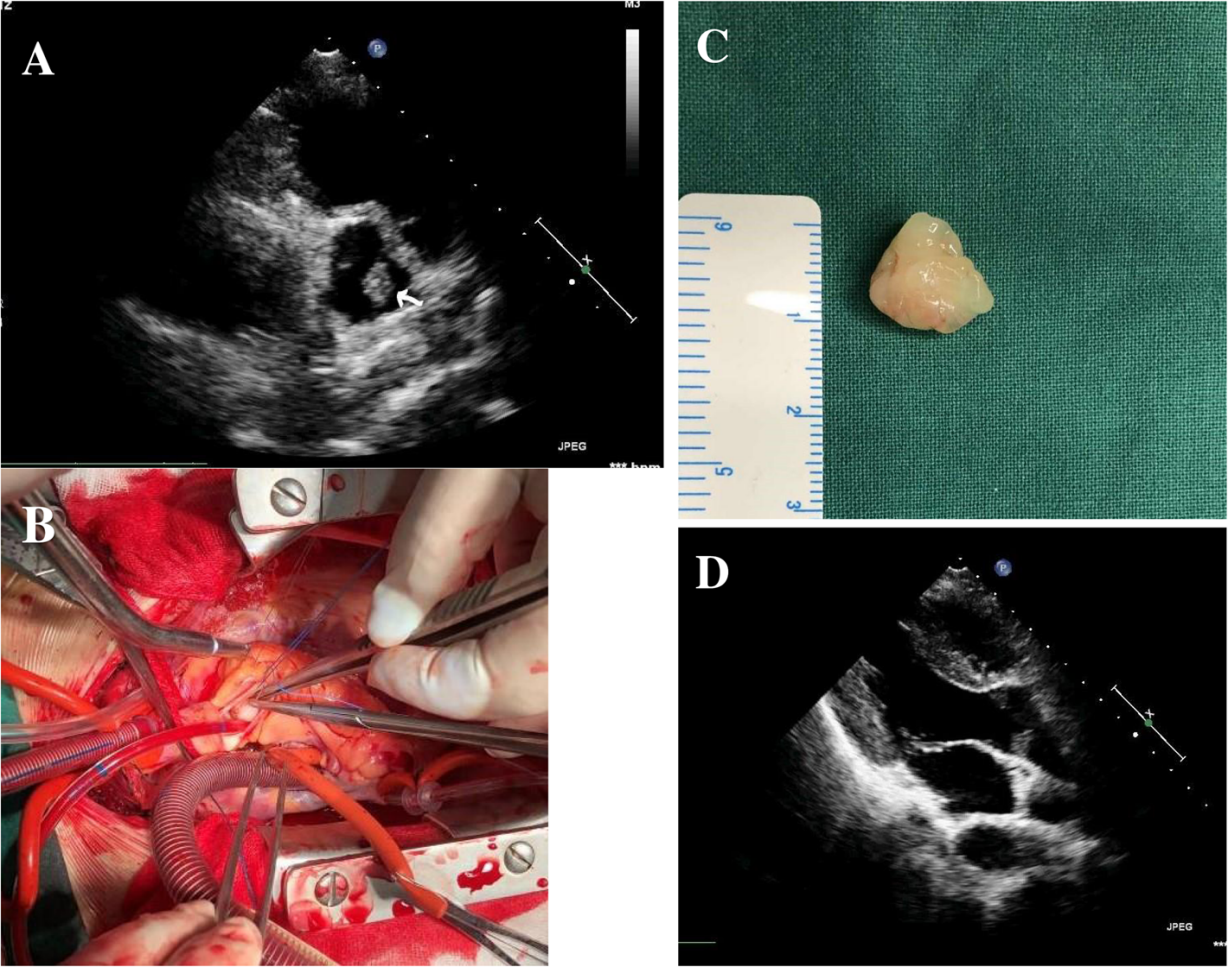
Panels a & d, pre/post operation echocardiography (white arrow-myxoma), b-aortotomy & c –myxoma
